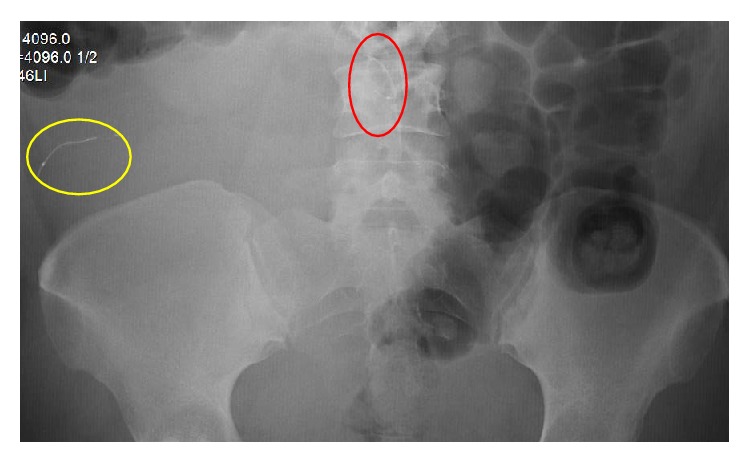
Patient Number 2, postpartum pelvic X-ray 10/13/15: Essure (circled in yellow) in the right upper quadrant around the liver. The 2nd Essure insert (circled in red) is seen by lumbar vertebra.
